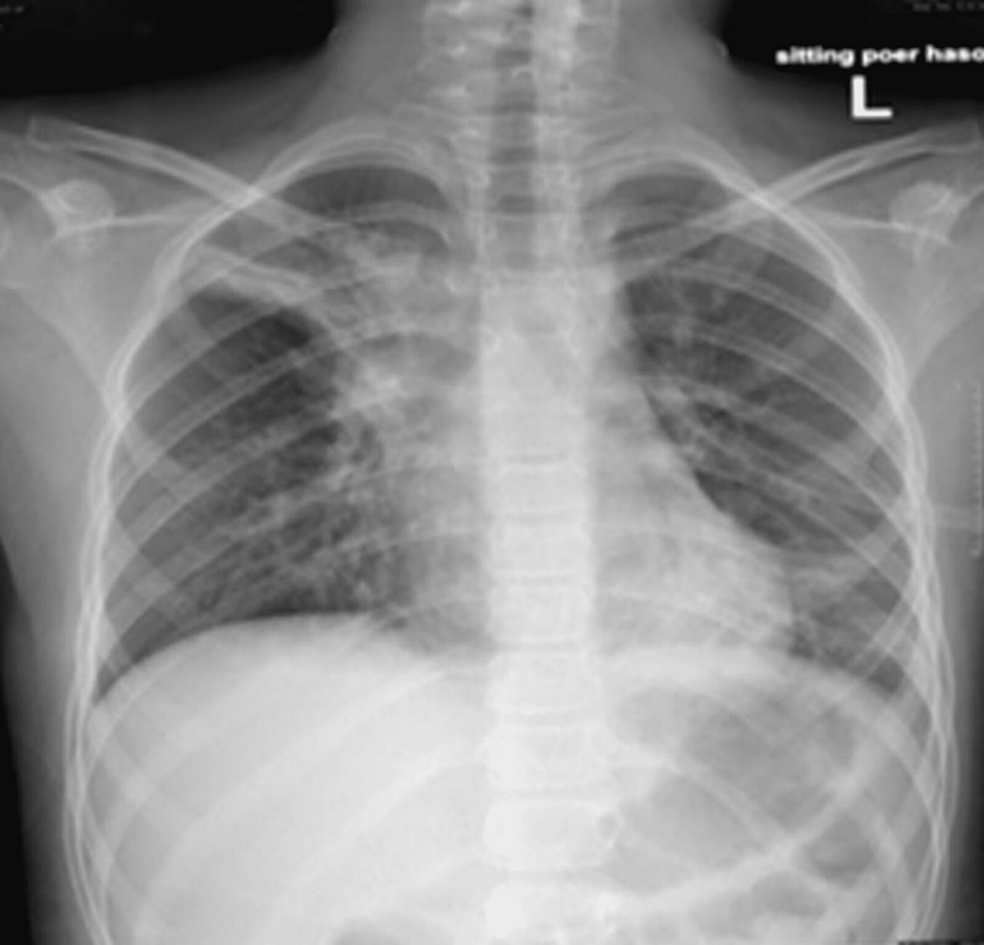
Chest X-ray showing bilateral peribranchial pneumonic infiltration, especially in the right upper zone, and subsegmental lung atelectasis
